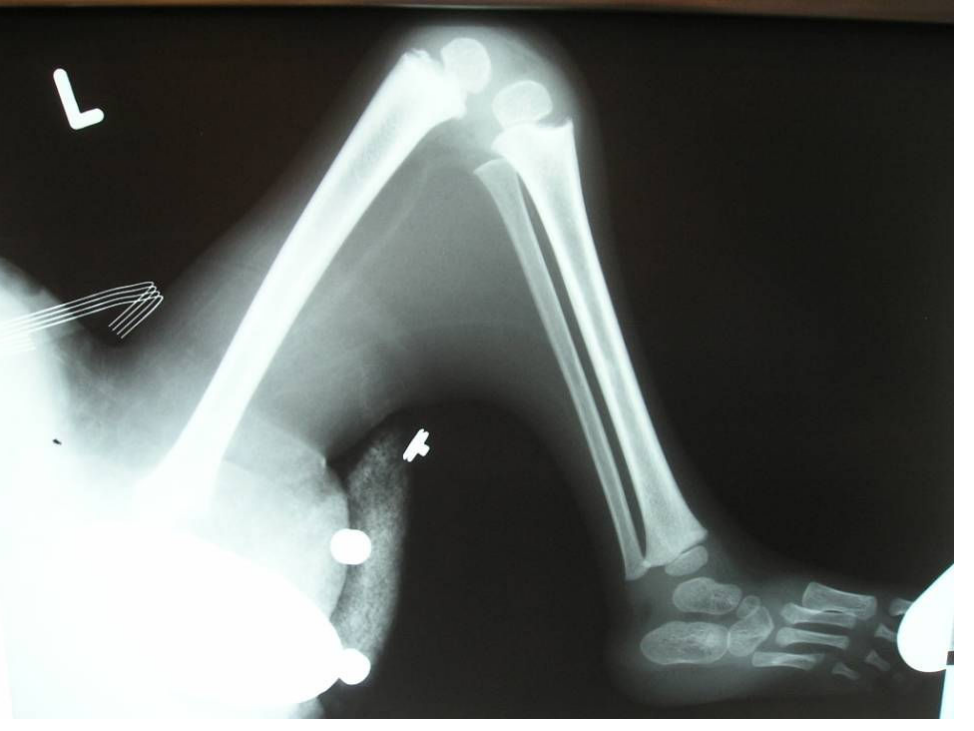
Lateral lower limb radiograph showed fixed deformity of the left knee associated with soft tissue web behind the knee extends more than halfway down the tibia to the ankle associated with oligodactyly associated with significant dysplasia of the tarsal bones, agenesis and hypoplasia of the metatarsals and agenesis of the fourth metatarsophalangeal and proximal phalanx.
